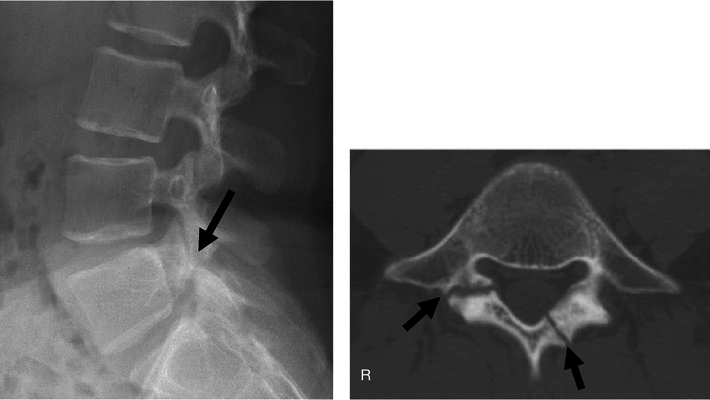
Left panel*: lateral plain X-ray shows a defect in the L5 lamina. *Right panel*: CT images show two fracture lines. One is seen in the right pars interarticularis (spondylolysis), and the other is seen in the left lamina (laminolysis)
